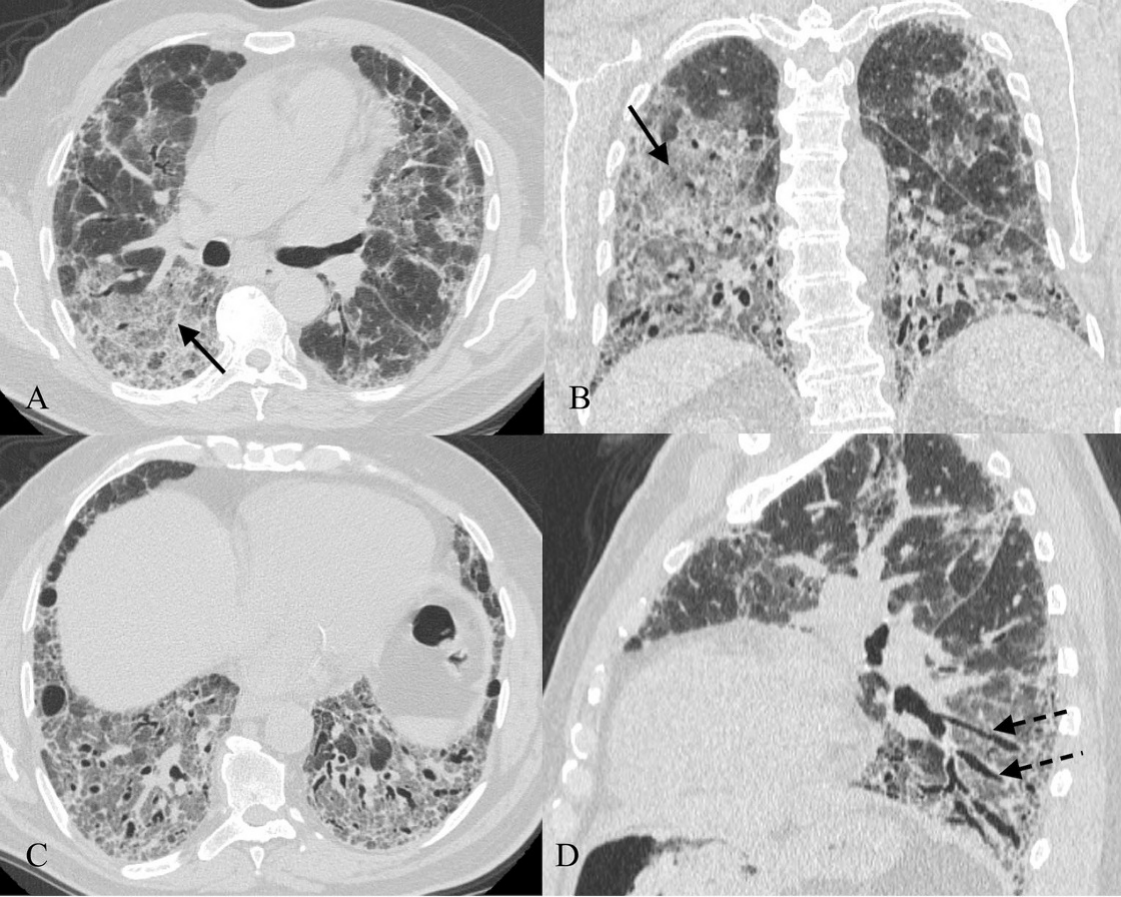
NSIP pattern in a 64-year-old man. HRCT features of NSIP include extensive ground-glass areas in the lung (black arrows) and traction bronchiectasis. This bronchiectasis frequently shows a parallel course through the lung, well depicted by sagittal reconstruction in D (black dotted arrows). There is no honeycombing in the lung. Cystic bronchiectasis is generally well documented by MPR images
